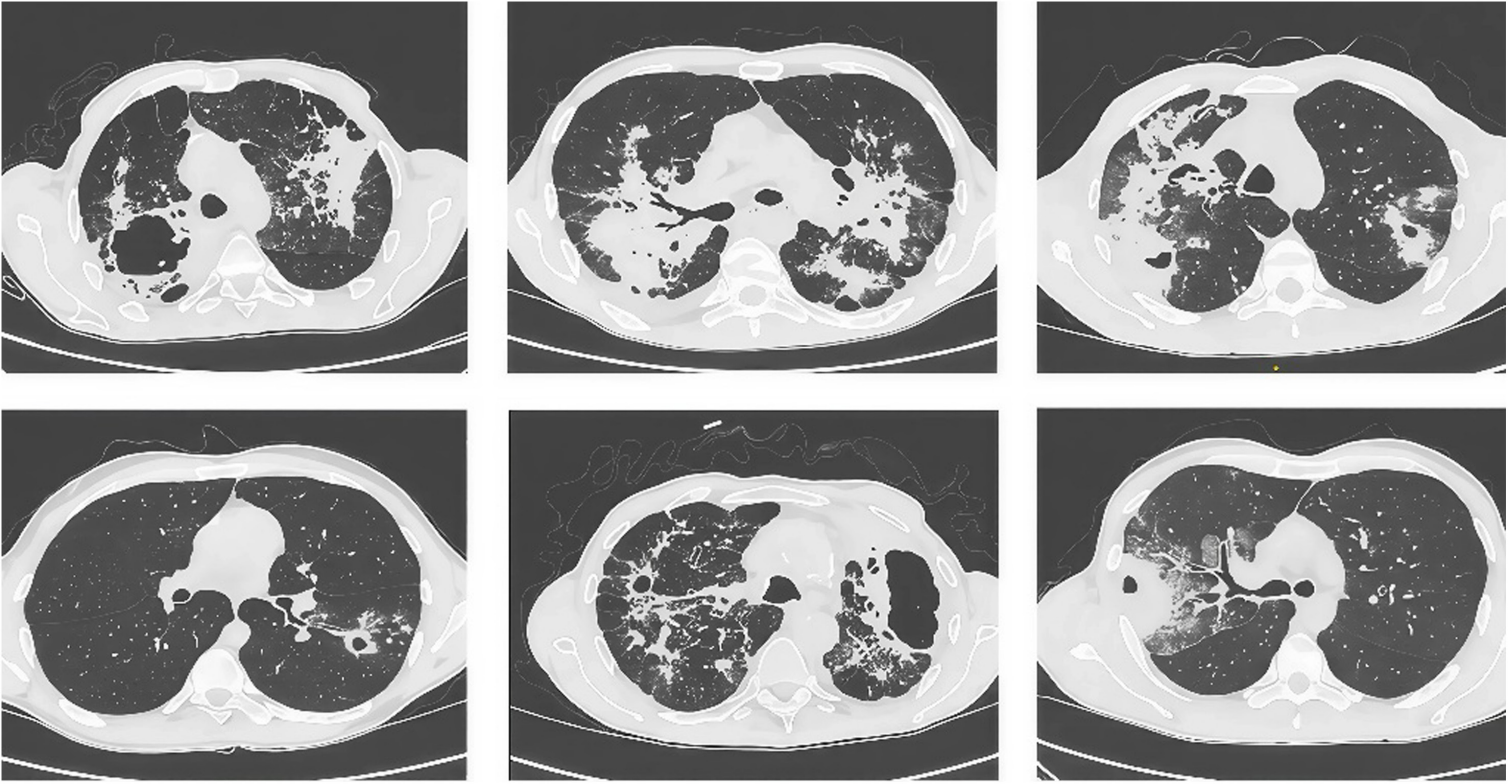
Examples of radiological findings in active tuberculosis visualized in HRCT.
